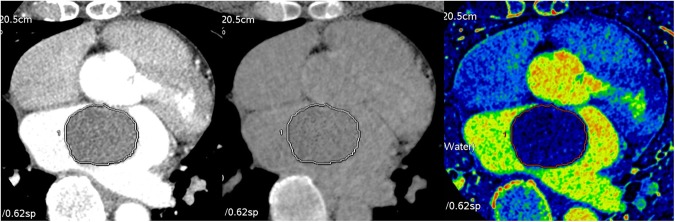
A representative image set for quantitative analysis of dual-energy CT of a cardiac tumor. Three modes of images were obtained using dual-energy CT: (A) a monochromatic 70 keV image representing the post-contrast CT image, (B) a MSI image representing the pre-contrast CT image, and (C) an iodine (water) image; A volume of interest covering the entire cardiac mass was drawn on the image of each mode on the identical level of the cardiac tumor.
